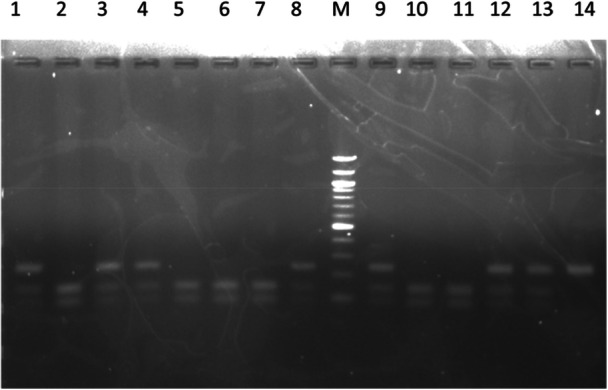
Restriction fragment length polymorphism analysis of rs3758391 C/T single‐nucleotide polymorphism (SNP) using NlaIII enzyme. 100‐bp ladder: Lane M; CC (241 bp): Lane14; TT (148 bp and 93 bp): Lane 2, 5, 6, 7, 10, 11; CT (241 bp, 148 bp, and 93 bp): Lane 1, 3, 4, 8, 9, 12, 13.
